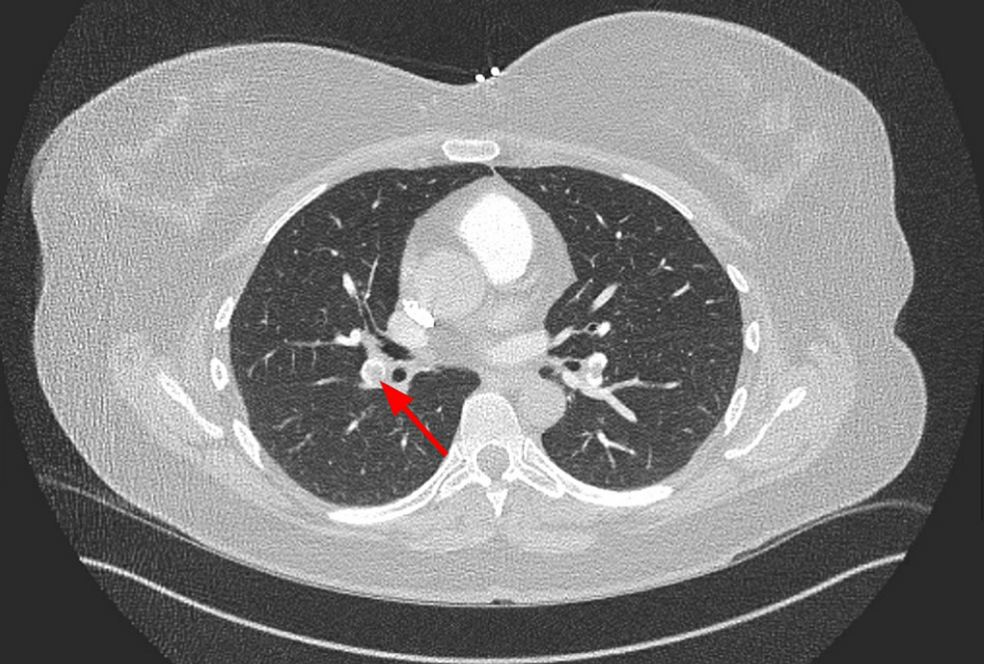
Redemonstration of thrombus in the right inter-lobar artery (red arrow) branching out from right pulmonary artery on CTA. CTA, computed tomography angiography
